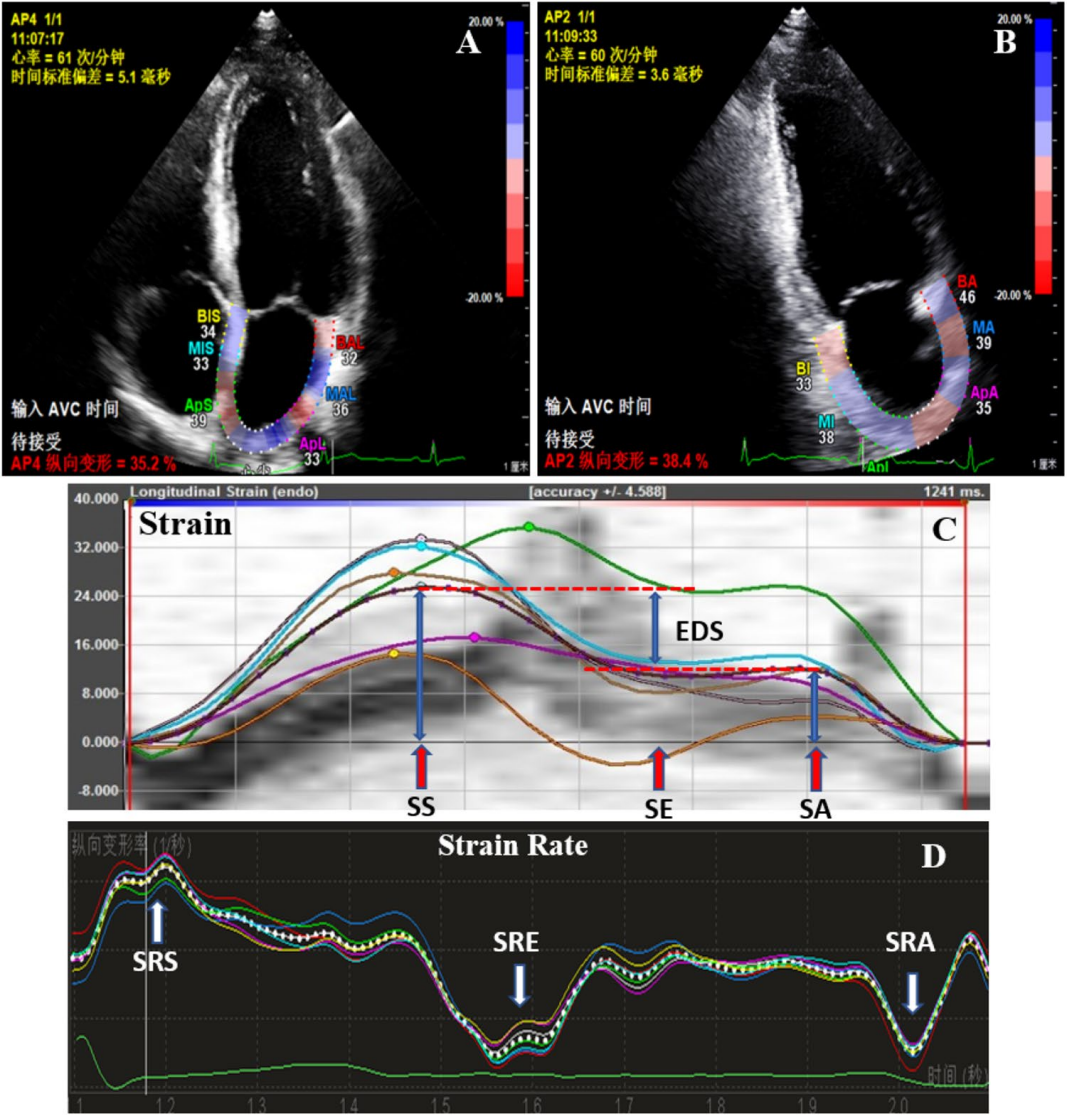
(A) Apical 4 chamber view; (B) Apical 2 chamber view; (C) Strain curve: The longitudinal LA strain curve had a positive systolic peak (SS), an early diastolic plateau (SE), and a late diastolic peak (SA); (D) Strain rate curve: the longitudinal LA strain rate curve had a positive systolic peak (SRS), an early negative peak at early diastole (SRE), and a late negative peak at late diastole (SRA). SS and SRS represented the LA reservoir function, EDS (= SS − SA) and SRE reflected the LA conduit, and SA and SRA were the indices of the LA booster function.
